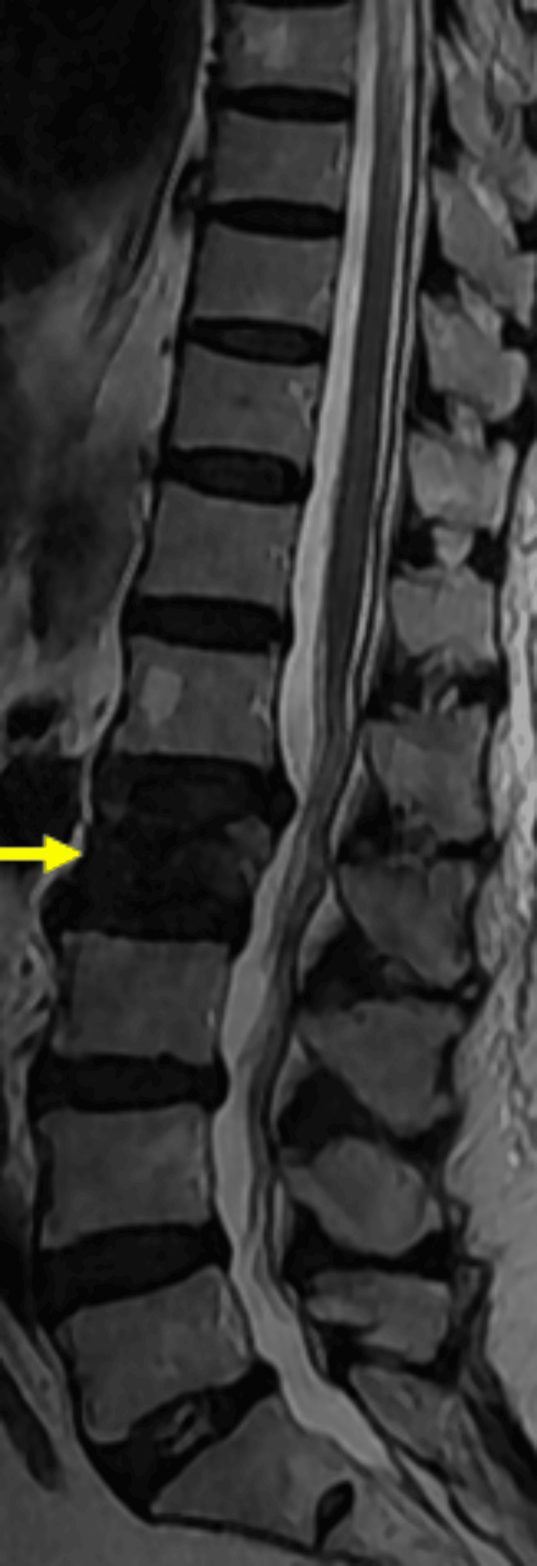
Pre-procedure sagittal T2-weighted magnetic resonance imaging of the thoracolumbar spine demonstrating an acute osteoporotic compression fracture of the L2 vertebral body, characterized by loss of vertebral height and altered marrow signal intensity (yellow arrow).
